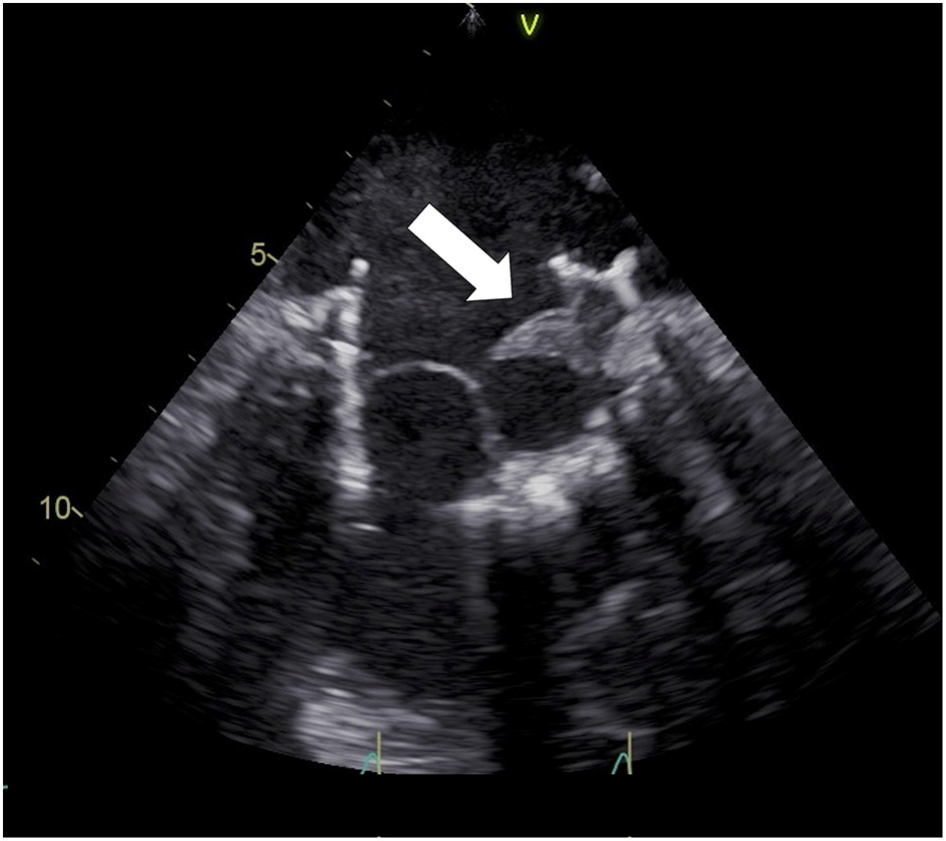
Transesophageal echocardiography showing Tiara (Neovasc) anterior leaflet thrombosis.
